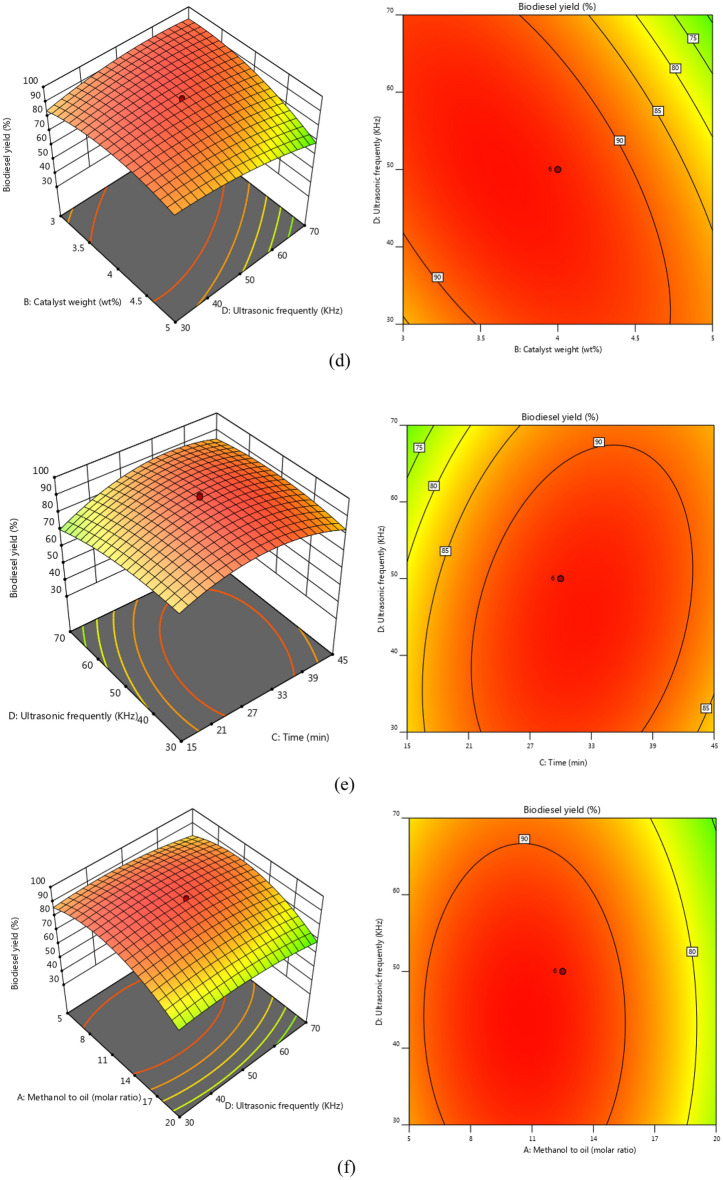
The 3D surface and 2D contour plots of interaction between (a) catalyst weight and methanol to oil molar ratio, (b) reaction time and methanol to oil, (c) catalyst weight and reaction time, (d) ultrasonic frequency and catalyst weight, (e) ultrasonic frequency and reaction time, (f) Ultrasonic frequently and methanol to oil.
